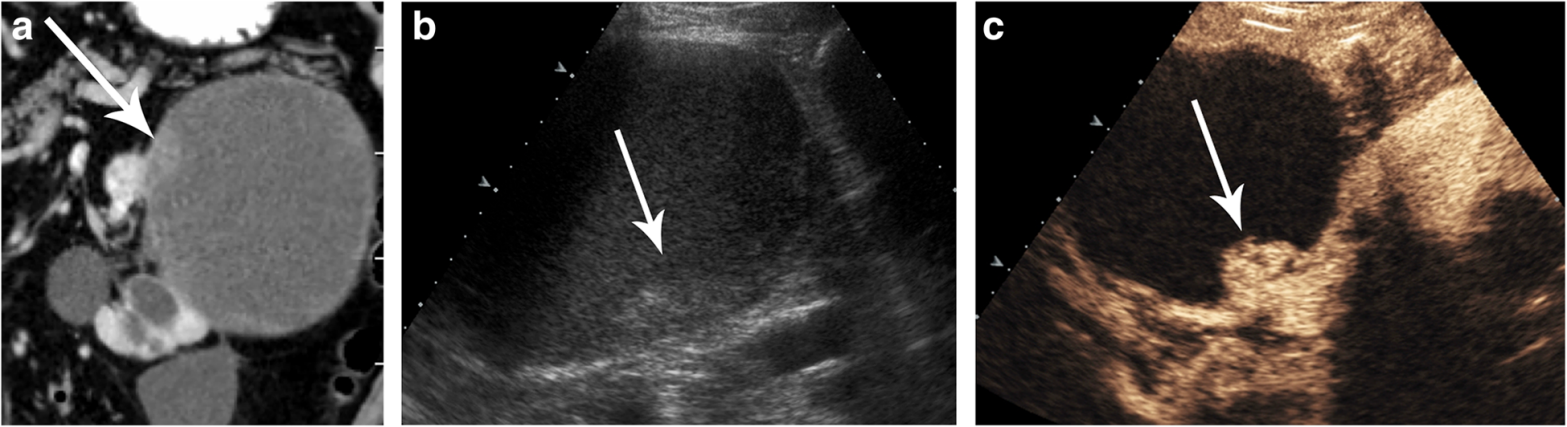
Contrast-enhanced US image showing a cystic renal lesion in the wall of the left kidney suspected of being a solid lesion. a Coronal contrast-enhanced CT showing a cystic renal mass with a hard-to-define image in its wall (arrow). c Unenhanced and (d) contrast-enhanced US clearly depicting enhancement on this solid nodule (arrows) in the cyst wall, leading to the lesion being defined and classified as a Bosniak II lesion
